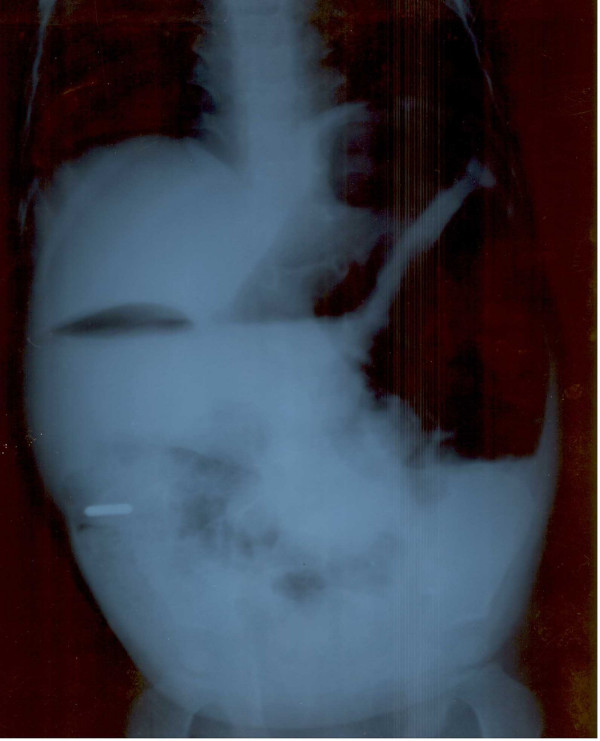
Plain radiograph of the abdomen showing the metallic foreign body in the right lower quadrant, the presence of a 'double bubble sign', and a few dilated loops of small bowel in the left upper quadrant.
